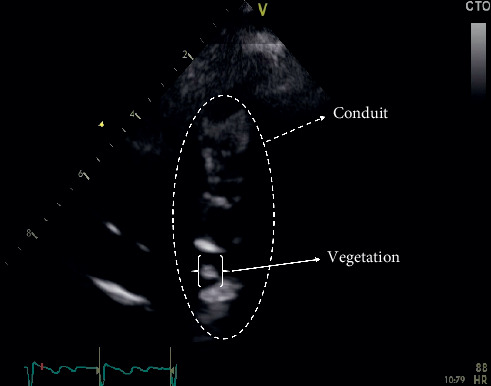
Transthoracic echocardiogram (TTE): a calcified vegetation, 4.0 mm × 3.0 mm in size, with independent mobility, was identified inside the conduit.
